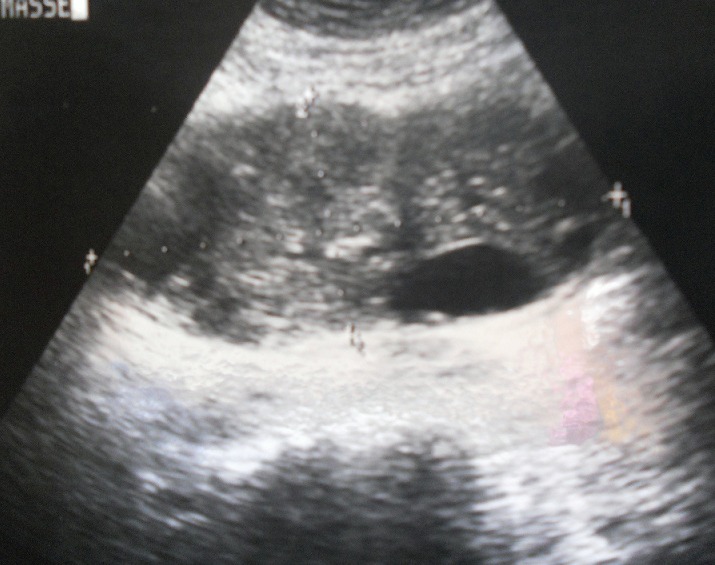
Abdominal ultrasonography (USG) showed a heterogeneous intraperitoneal mass
